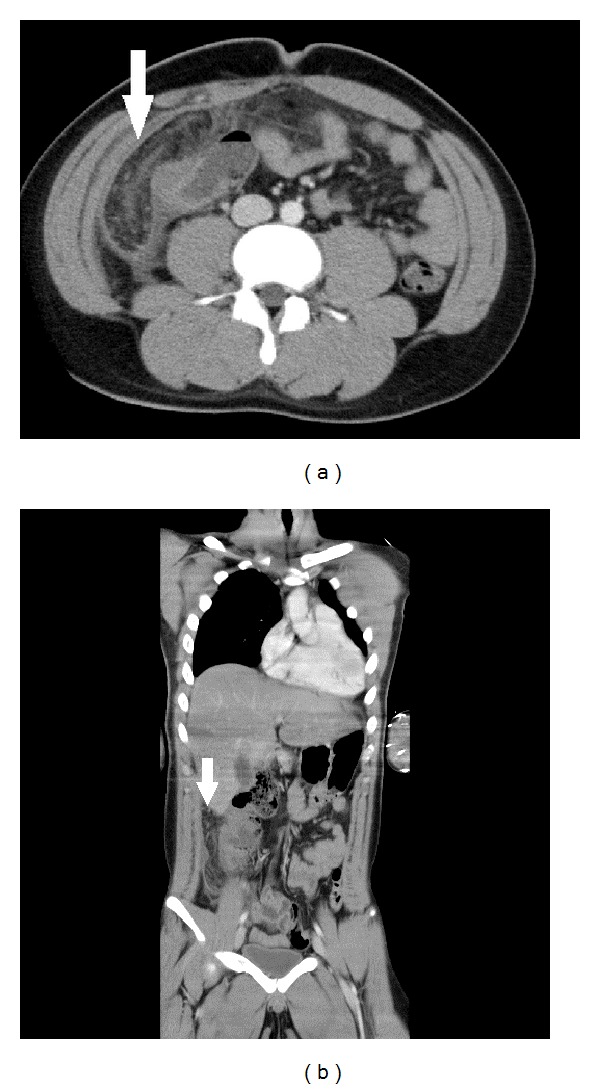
Contrast-enhanced abdominal CT. (a) The fat density of the greater omentum has increased (arrow), a finding that is compatible with a diagnosis of omental infarction. (b) The greater omentum has moved into the right paracolic gutters. A small amount of peritoneal fluid is detected around the omentum.
